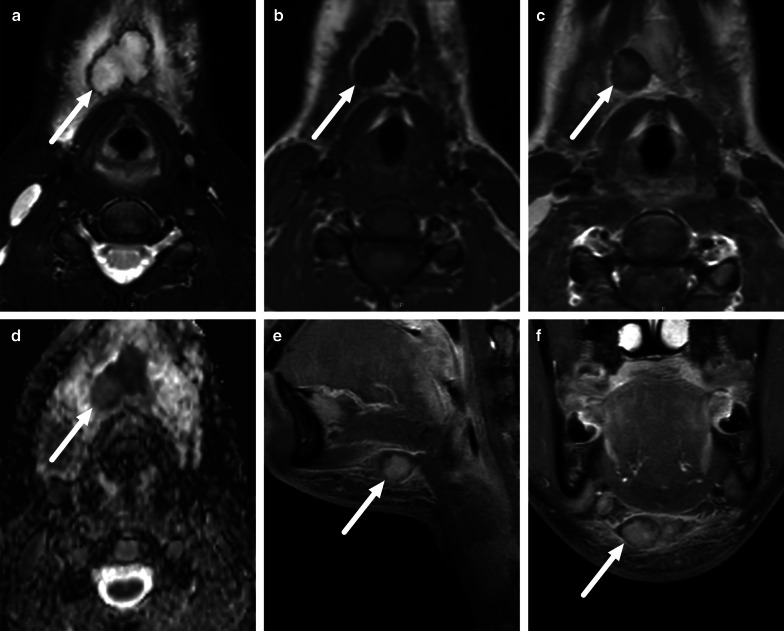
Necrotic lymphadenitis (arrows) misdiagnosed as suppurative lymphadenitis in a teenager. Lymphadenitis is seen on the axial fat-suppressed T2-weighted image (a) and poor enhancement on the post-contrast T1-weighted image (c) compared with the pre-contrast image (b). ADC shows restricted diffusion (d). The finding was misinterpreted as suppurative lymphadenitis; however, at later time points, delayed enhancement is seen (e, f), ruling out suppuration. Surgery found necrosis, but no pus. Image modified from ref. [8]
